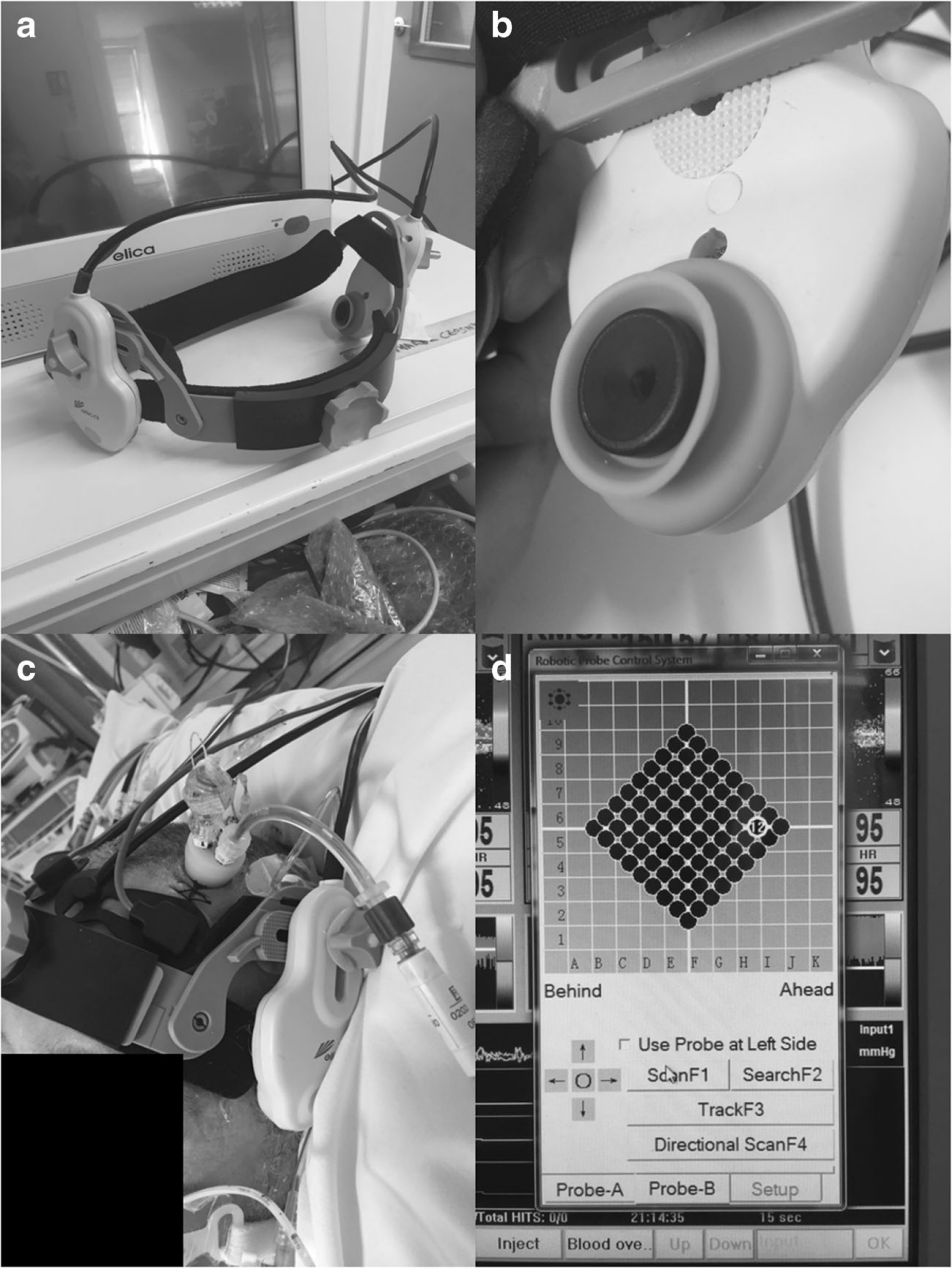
Example of recording set up for ICP and robotic TCD. ICP intracranial pressure, TCD transcranial Doppler. a Displays Delica robotic TCD headframe with two robotic drives mounted to a ratcheting headband system. b Displays close up image of TCD probe encased in robotic drive. c Example of set up for multi-modal monitoring with left frontal triple bolt (ICP, microdialysis and brain tissue oxygen monitoring), bifrontal near infrared spectroscopy, robotic TCD. d Delica EMS 9D TCD program display, showing automatic flow velocity sampling algorithm, producing a square grid of sampled insonation positions. The system insonates at multiple sites, finding the area with superior signal quality)
